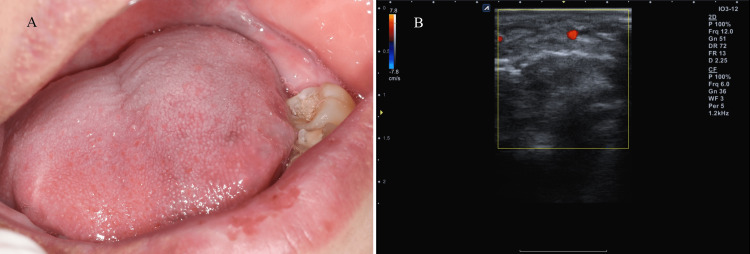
Three-month follow-up after sclerotherapy (A) Clinical appearance (B) Ultrasonography doppler image
